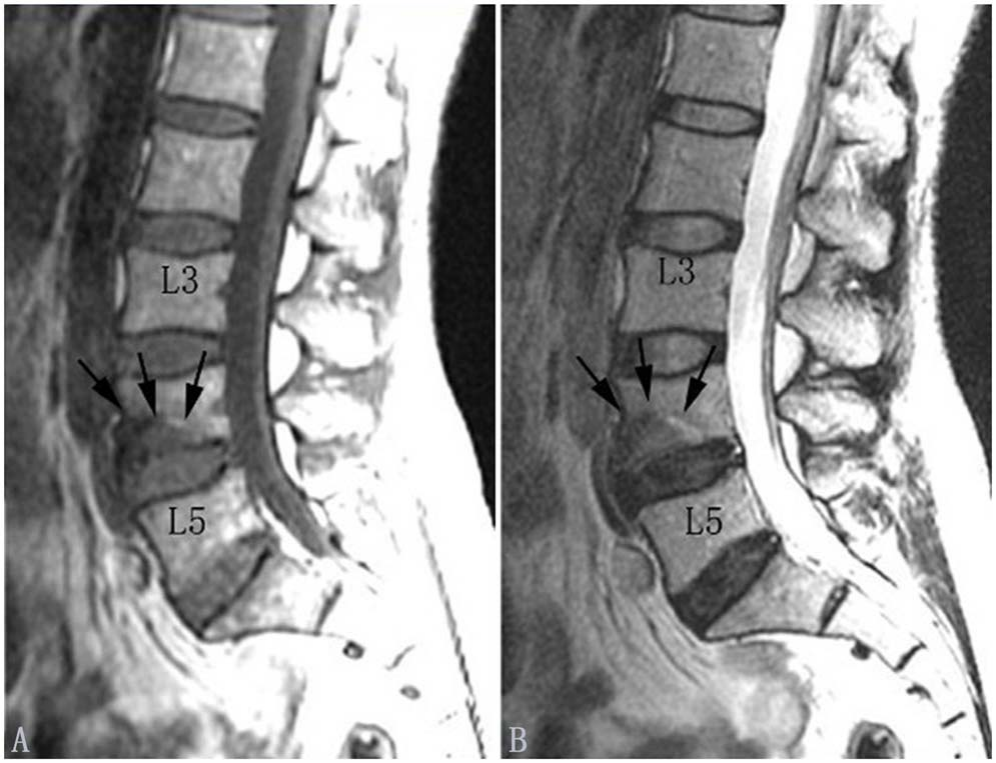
Modic type III changes in the lumbar spine (indicated by arrows). Modic type III changes appear hypointense on T1-weighted (A) and hypointense on T2-weighted images (B).
